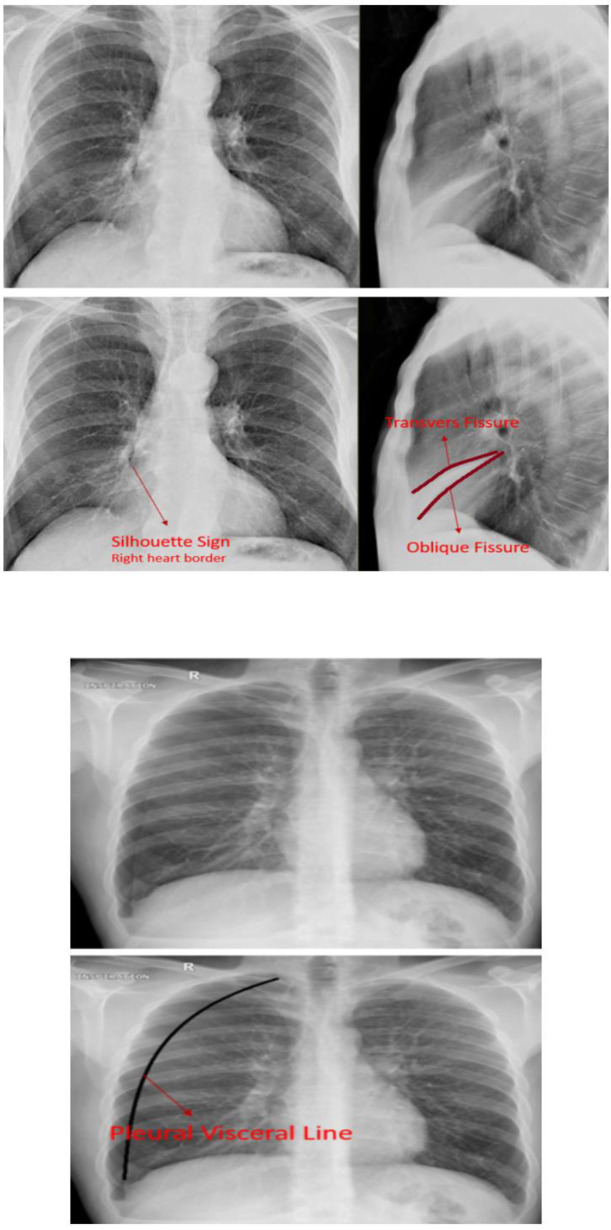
Examples of electronic content designed for student instruction, including identification of radiograph abnormalities, upper: right middle lobe atelectasis, lower: pneumothorax.
